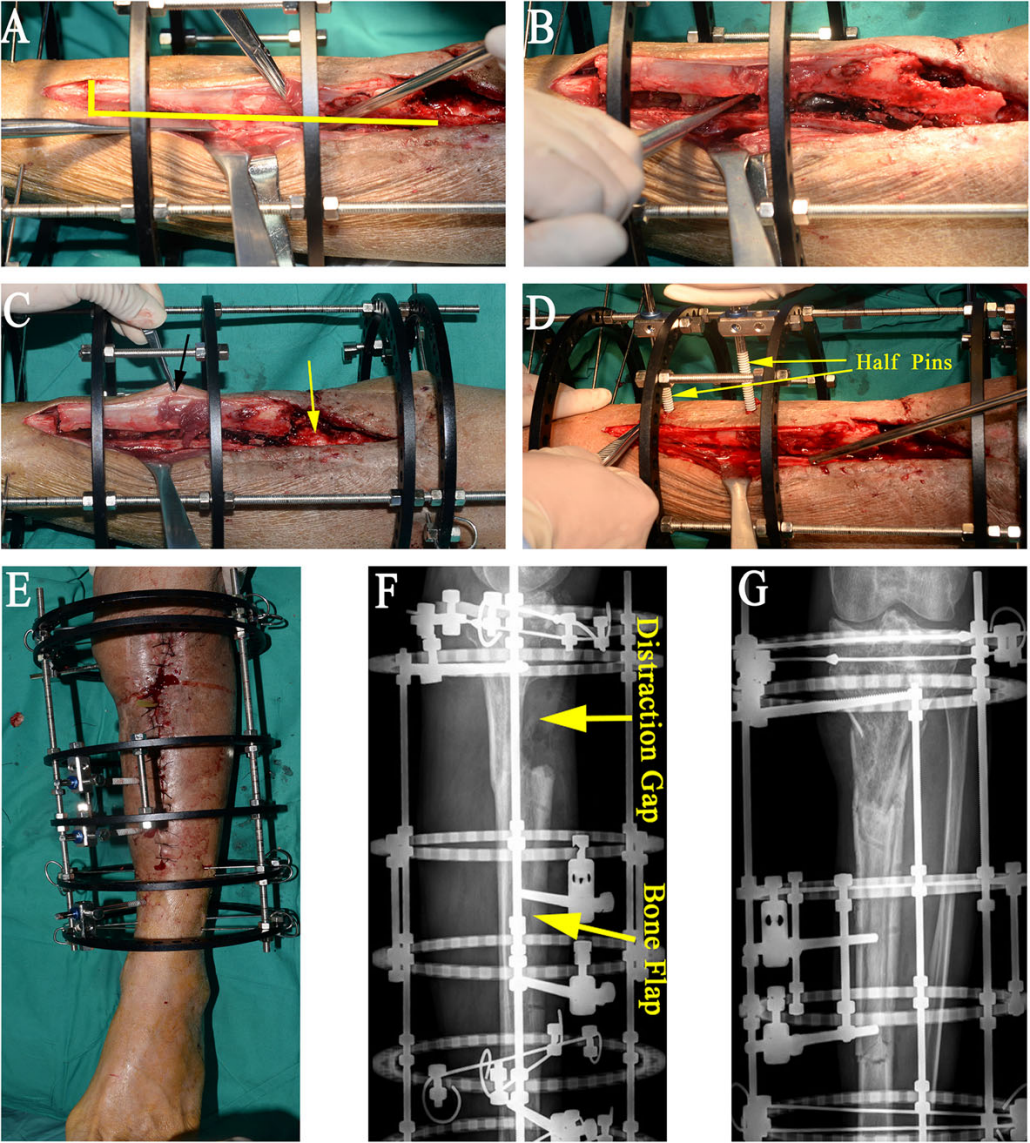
Application of external fixator and L-shaped osteotomy. a The holes drilled by Kirschner wires form an L-shaped configuration (yellow line), and osteotome is used to separate the bone flap. b The bone flap is separated from the posterior half of tibia. c The bone flap is not separated from the soft tissue it attaches to, thus being provided with good blood supply from the anterior and lateral soft tissue (black arrow), and remaining posterior cortex after debridement and sequestrectomy keeps the periosteal attachment intact and also ensures a good blood supply (yellow arrow). d Two 5.0-mm half pins are used for unilateral cortical fixation of the bone flap. e The incision is closed with drainage tube. f, g Immediate postoperative latera and anteroposterior radiographs
